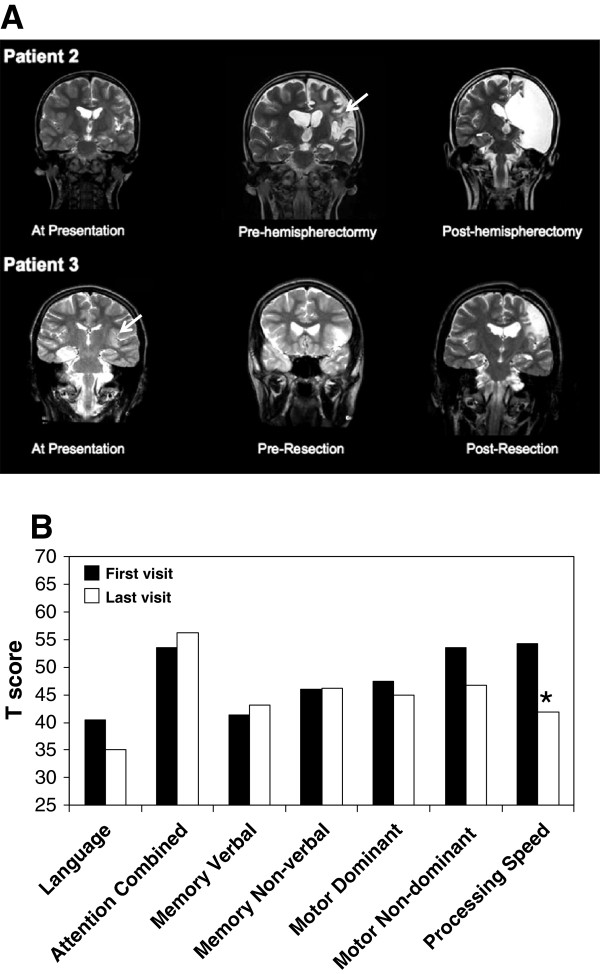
Neuroimaging and neuropsychological profiles in Rasmussen’s encephalitis (RE). (A) In patient 2, coronal MRI T2-weighted images at presentation revealed mild left hemisphere atrophy and cortical thinning, 3 years after presentation revealing profound left hemispheric atrophy and hyperintensity of white matter (arrowhead), and post-hemispherectomy. Similarly, in patient 3, increased white matter signal was evident at initial presentation but actually resolved after corticectomy. (B) Median T scores (population mean 50; standard deviation 10) in seven neuropsychological domains for patients 2, 3, and 4. Median first assessments are represented by black bars and the median last assessment as white bars. Mann–Whitney *U* test, P* <0.05. MRI, magnetic resonance imaging; RE, Rasmussen’s encephalitis.
